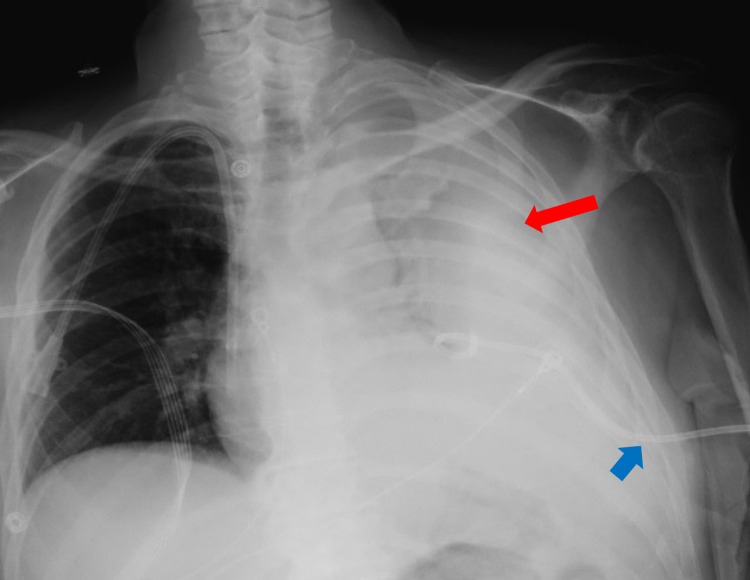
Chest X-ray showing complete opacification of the left hemithorax (red arrow) after chest tube insertion (blue arrow).
